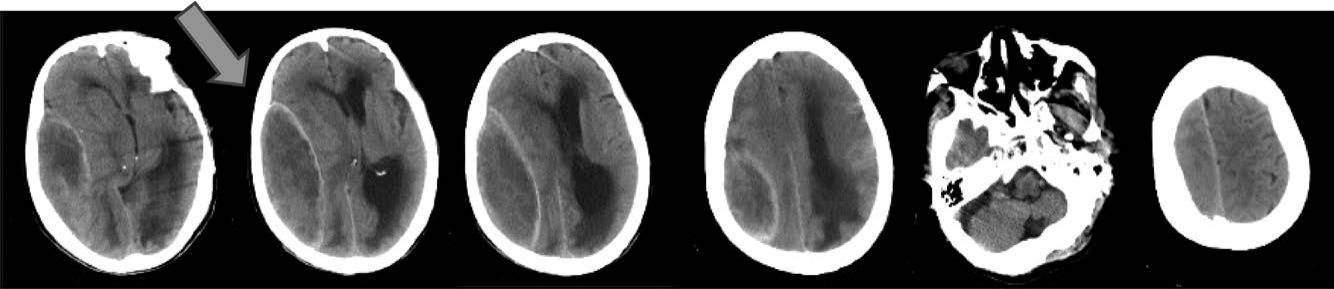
Preoperative noncontrast CT scan of the patient’s head. Fusiform isodense/slightly dense shadow was seen under the right temporoparietal occipital cranial plate, which was considered a subacute epidural hematoma. The maximum layer was 117 mm×42 mm, and the thickness of the 10 mm layer covered 7 layers. The right lateral ventricle was compressed. The midline of the brain shifted to the left. CT = computed tomography.
